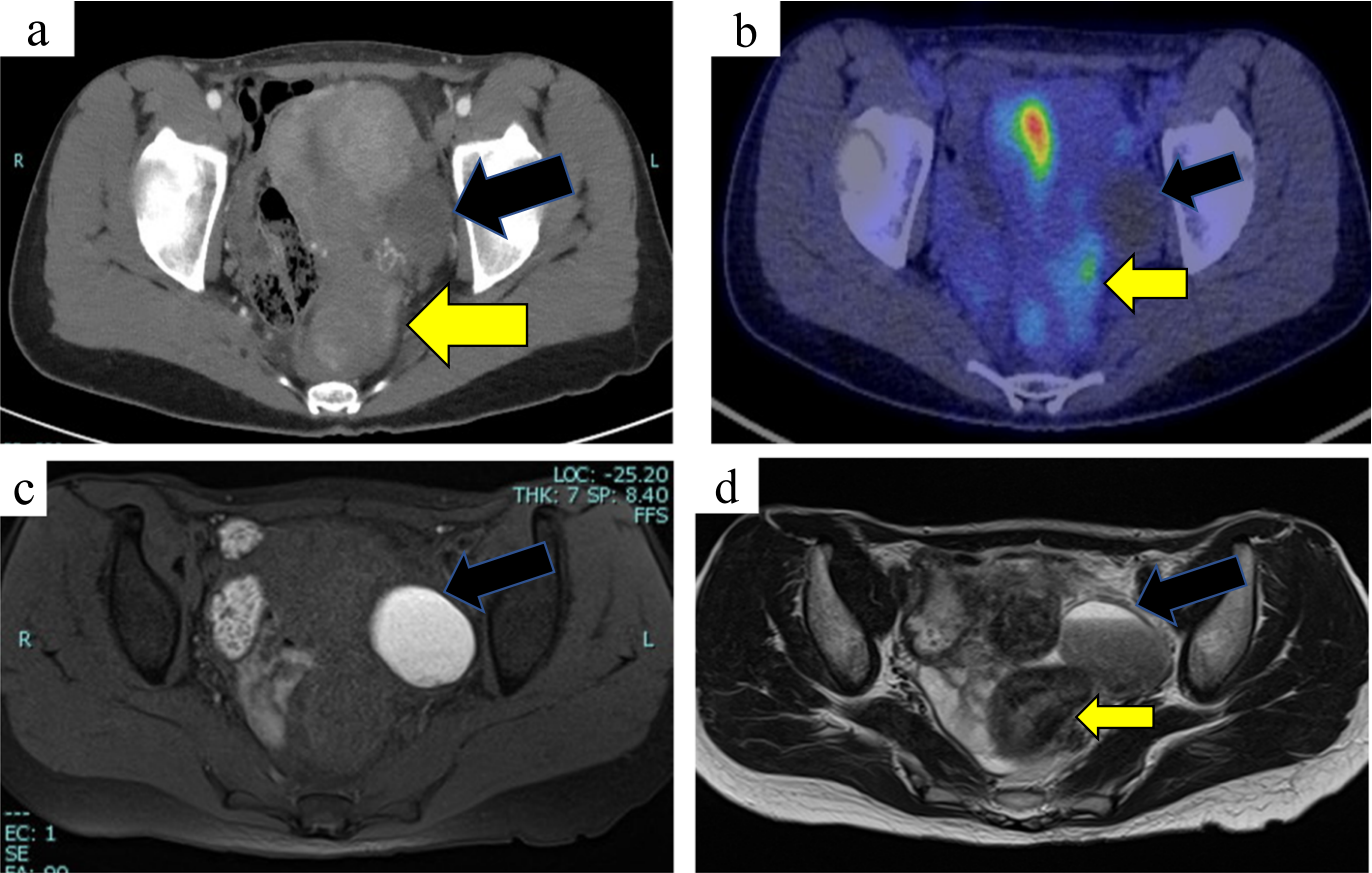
Computed tomography and magnetic resonance imaging findings. Computed tomography revealed thickening of the rectal wall (yellow arrow) and a left ovarian cyst (black arrow). Positron emission tomography-computed tomography revealed a slight increase in fluorodeoxyglucose uptake at the wall of the rectum. Magnetic resonance imaging revealed a left ovarian chocolate cyst and a thickened rectum wall
